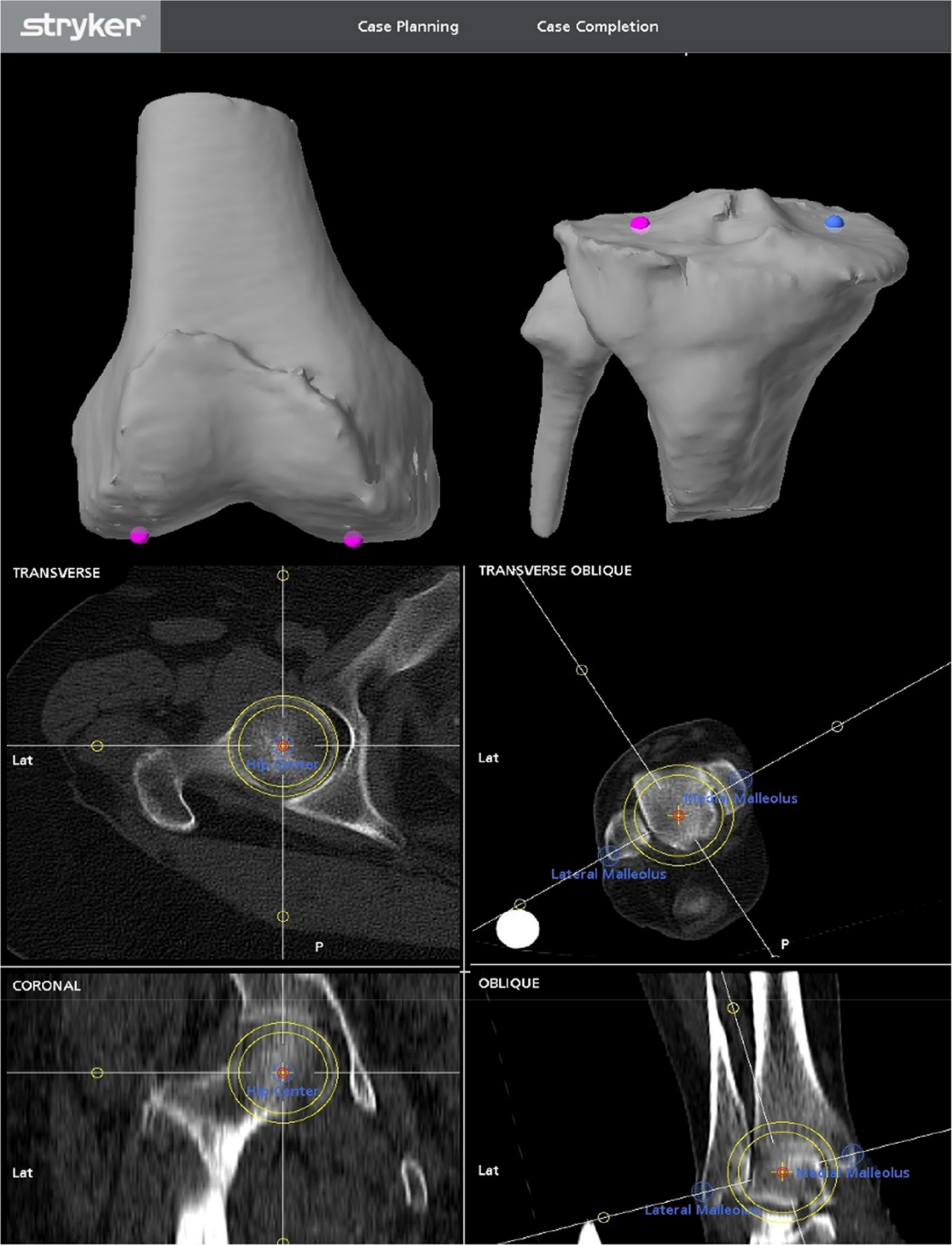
Mako computed tomographic robotic planning of alignment landmarks. CT images and 3D reconstructions were based on preoperative CTs within the Mako software, demonstrating distal femoral and proximal tibial landmarks and selection of the femoral head and ankle centre. Landmark acquisition was based on points at the most distal part of the condyles for the femur, and centre at 2/3 of the distance from the front of each plateau for the tibia, as demonstrated in the uppermost image
